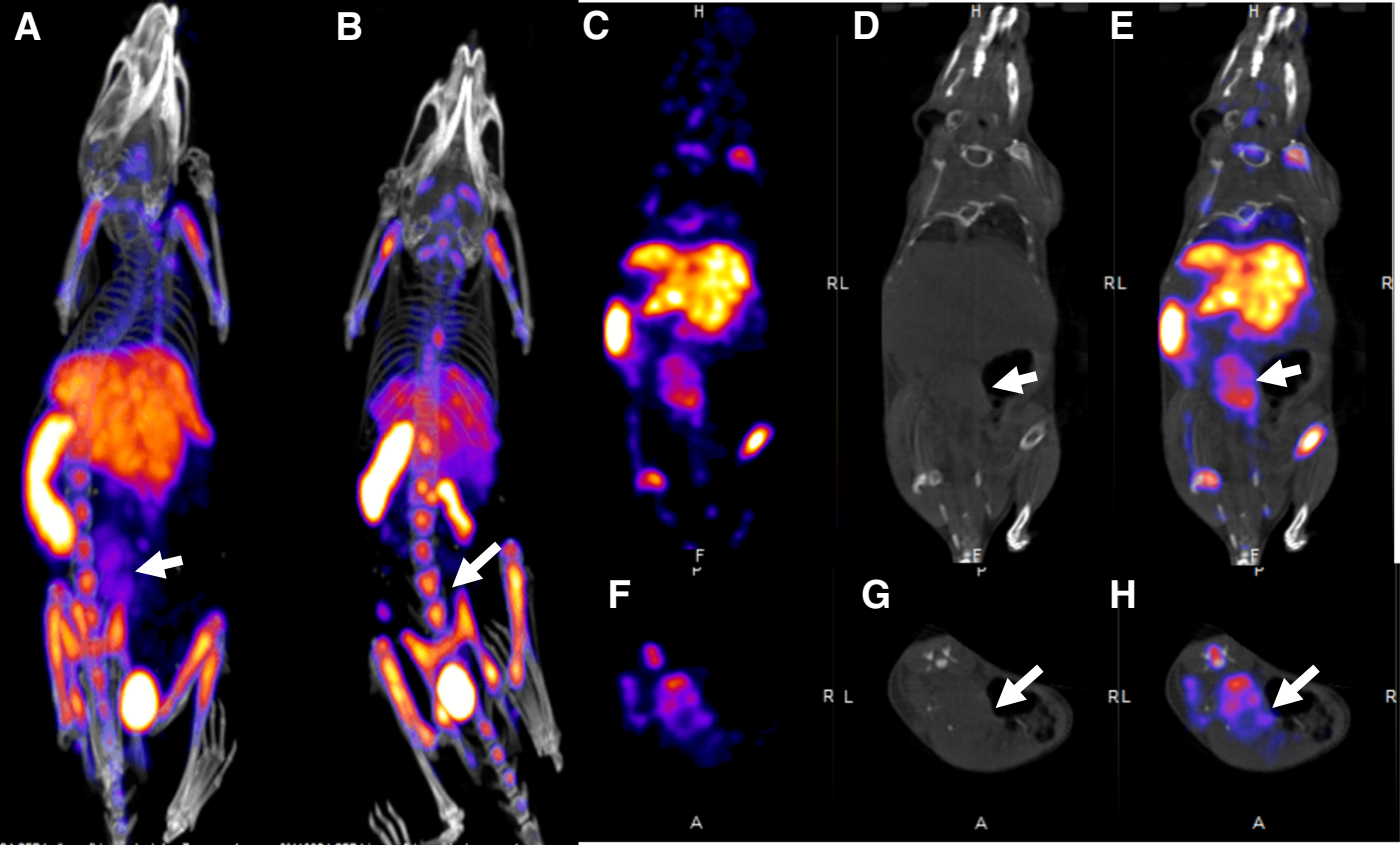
^99m^Tc-SER-4 nanoSPECT/CT imaging of Sn^+^ macrophages in heterotopic cardiac transplant model. Representative maximum intensity projection nanoSPECT/CT images of recipients with either a allogeneic or b syngeneic heart graft imaged 3 h post injection with ^99m^Tc-SER-4. CT, SPECT and fused images from coronal (c, d, e, respectively) and transversal (f, g, h, respectively) planes are also shown. *Arrows* indicate site of abdominal cardiac transplant with uptake of tracer in allogeneic heart graft that are absent in the syngeneic heart graft model
